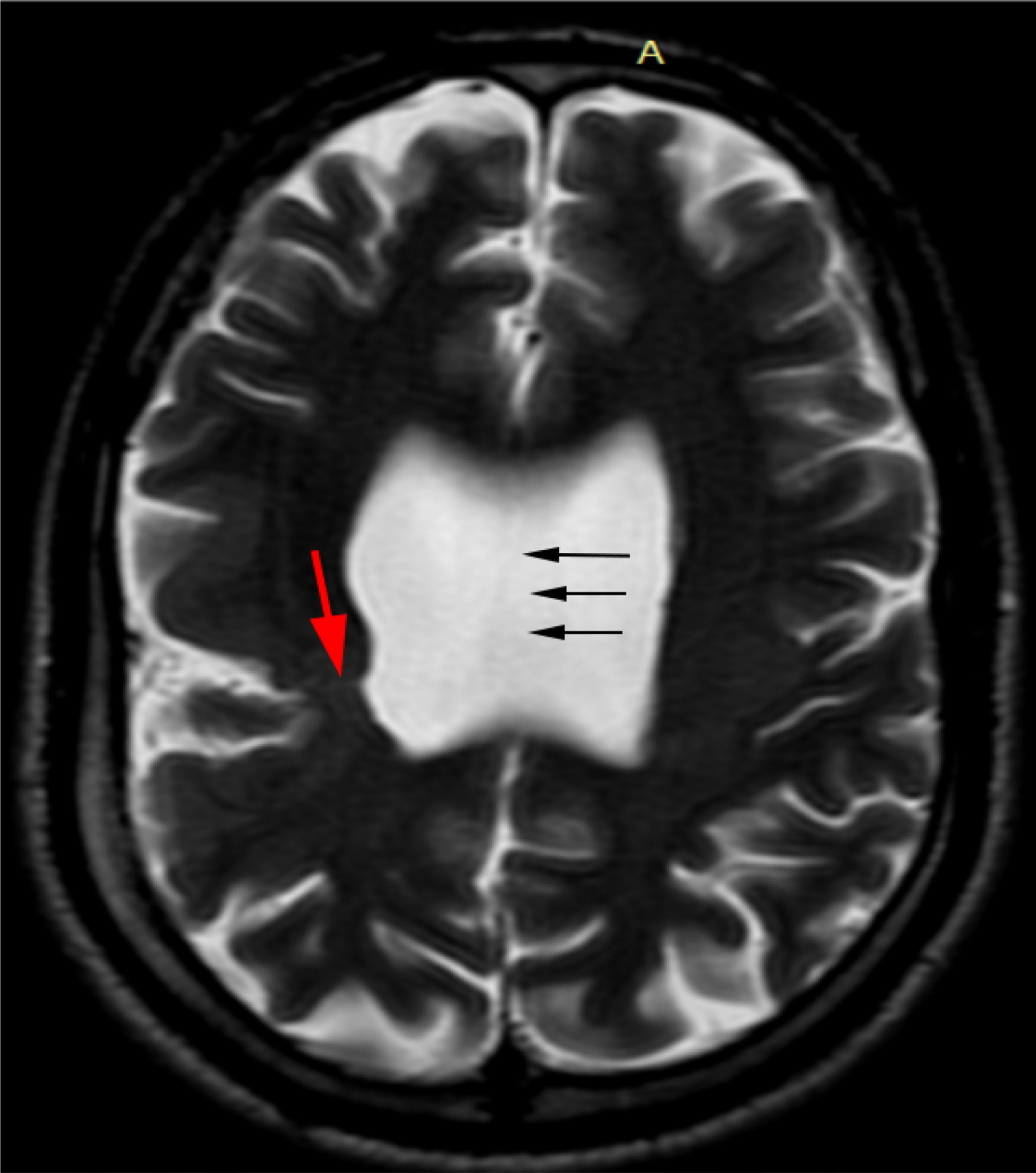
(At the level of lateral ventricle) T2W magnetic resonance imaging axial image shows a subtle T2 hyperintense curvilinear line (red arrow) communicating the lateral ventricle and subarachnoid space of right frontoparietal convexity. Black arrows denote the absence of the septum pellucidum resulting direct communication between the lateral ventricles and squaring off of the frontal horns.
